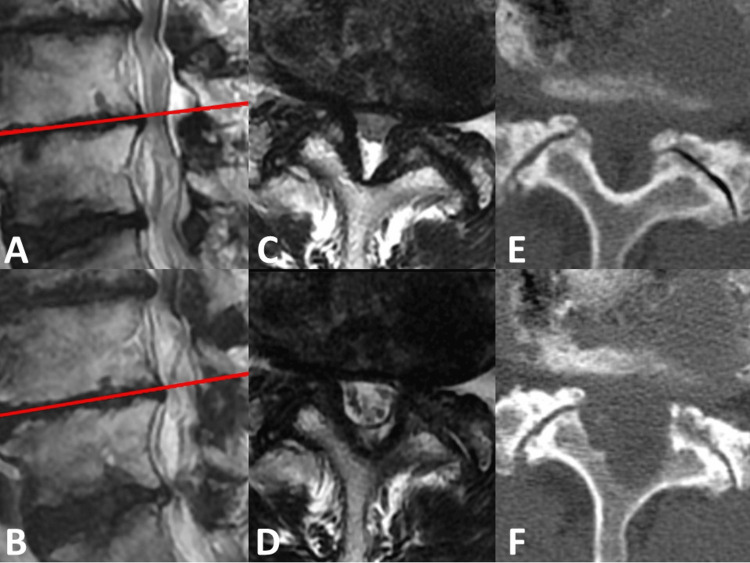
Bilateral decompression via unilateral approach (BDUA) performed by a 6.4-mm working channel FEL (A, B) Preoperative (A) and postoperative (B) sagittal T2-weighted magnetic resonance images (MRI) of the patient (78 years old, male) with L4/5 LSCS. The red lines indicate the scanning positions for axial MRI. (C, D) Preoperative (C) and postoperative (D) axial T2-weighted MRI. (E, F) Preoperative (E) and postoperative (F) axial computed tomography findings at the same scanning positions as MRI.
